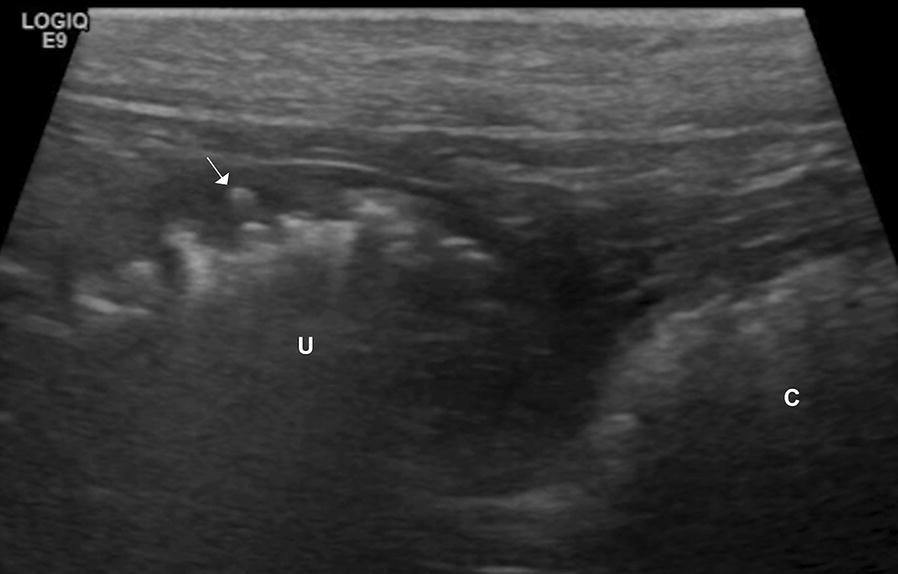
Ultrasound image in a sagittal plane of the caudal abdomen (left of the image is cranial). The uterus (U) is on the left of the image and the colon (C) is on the right. Note the uneven uterine luminal surface, with hyperechoic speckles in the wall (arrow), likely indicating infiltration of gas in the mucosa, indicative of emphysema or ulceration
